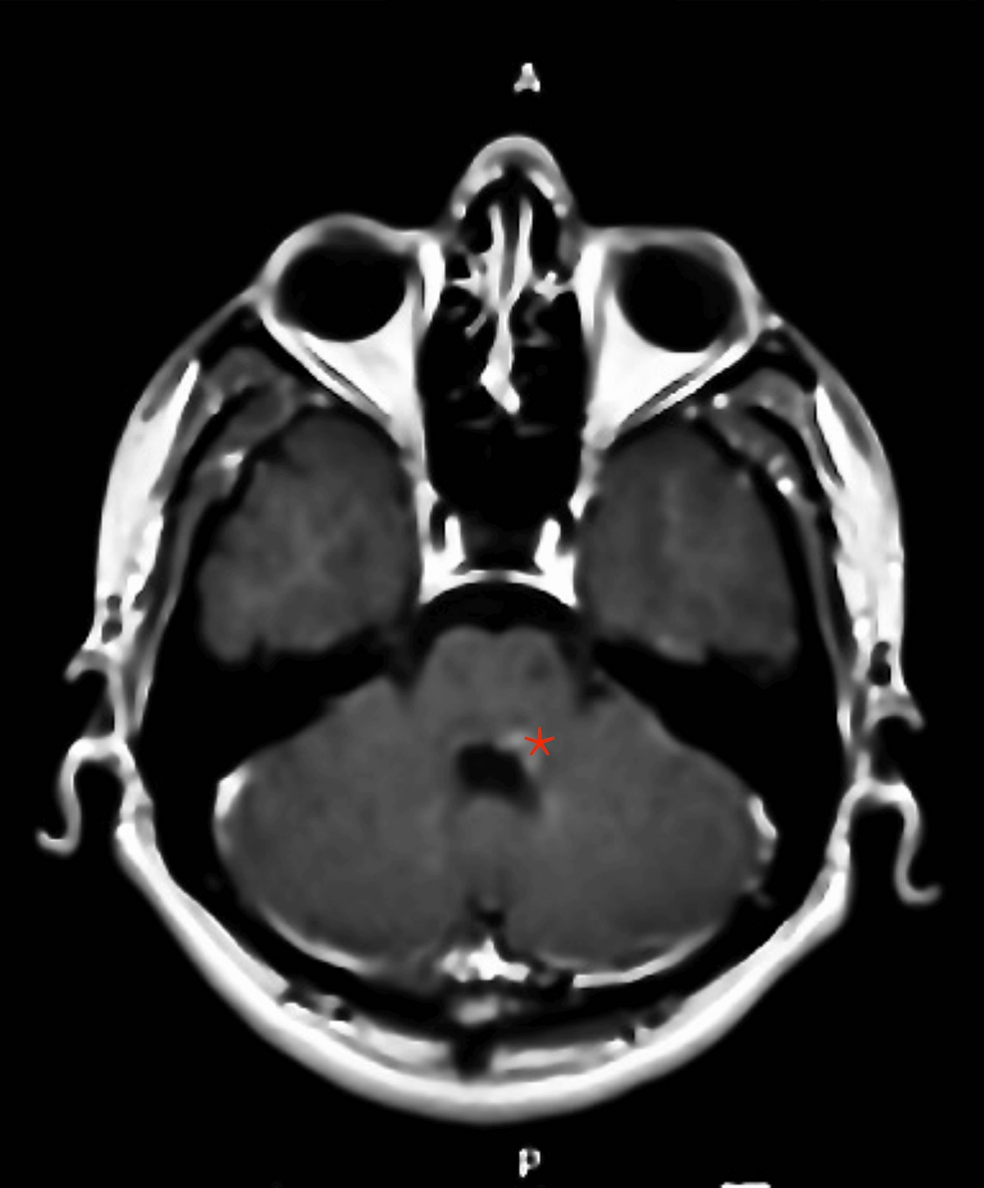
Contrast-enhanced MRI in axial view at the level of middle cerebellar peduncle Contrast-enhanced MRI displaying patchy abnormal enhancement on the left side of the dorsal pons, extending to the left brachium pontis (indicated by a red asterisk) MRI: magnetic resonance imaging
